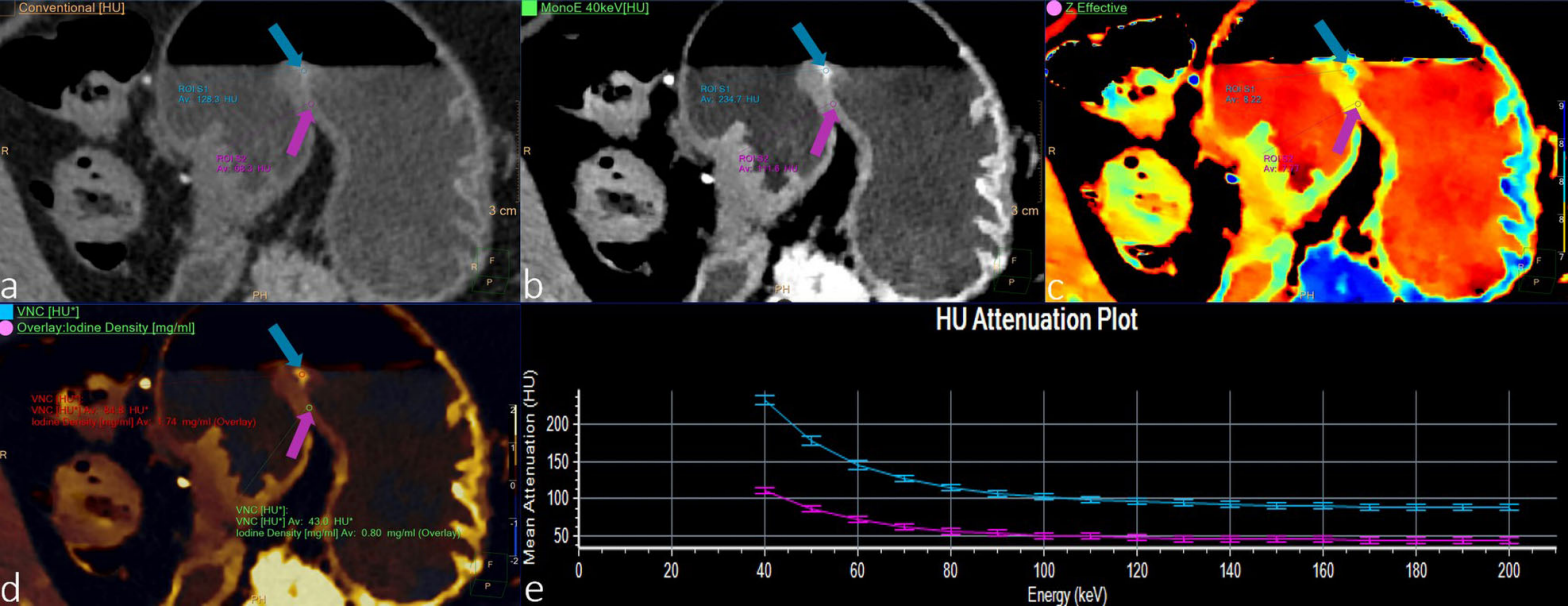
A 67-year-old female patient presented with recurrent abdominal pain, bloating, and acid reflux for six months. The pathological diagnosis was intramucosal carcinoma of the gastric horn. Arterial phase CT and postprocessing images: a conventional image; b 40 keV images; c
